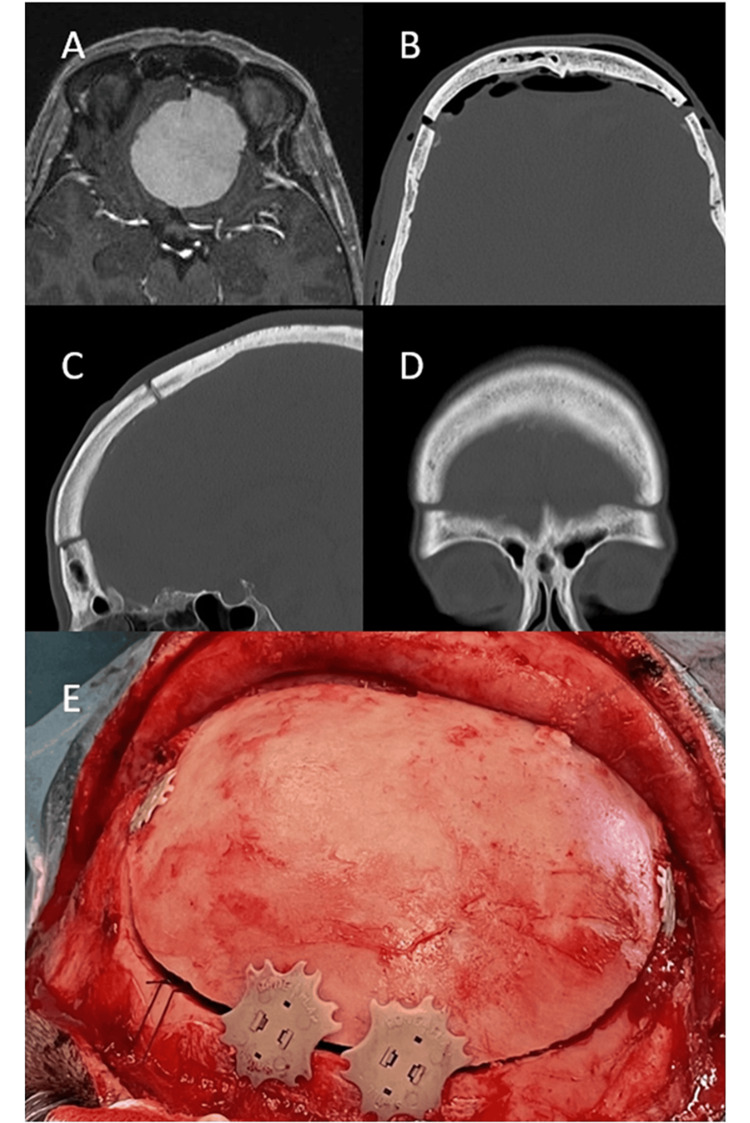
A 55-year-old woman undergoing resection of a meningioma on the olfactory groove (A) Pre-operative MRI image: axial view weighted in T1 with gadolinium showing a frontal basal meningioma. (B) Twenty-four-hour post-operative CT scan image: axial view of the bone. (C) Four-month post-operative CT scan image: sagittal reconstruction view of the bone. (D) Four-month post-operative CT scan image: coronal reconstruction view of the bone. (E) Surgical view after tumor resection and fixation of the bone flap with Cranial LOOP™.
